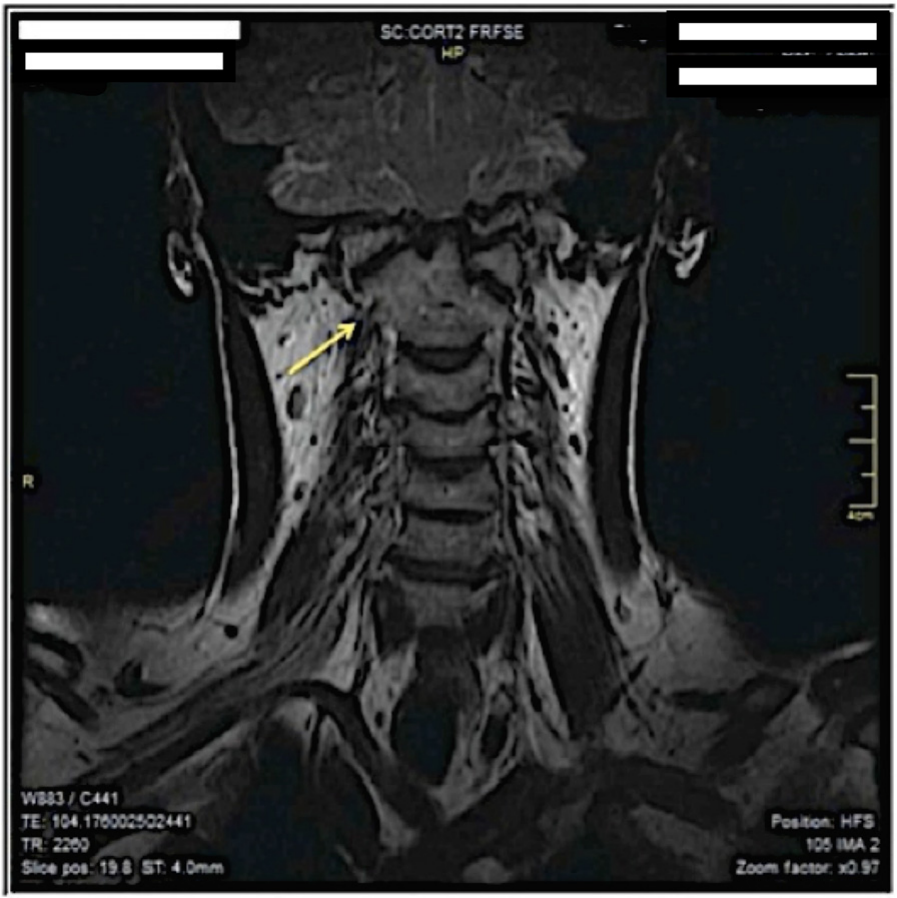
Coronal T2-weighted MRI. The MRI showing the cranial-cervical malformation with a subtotal fusion of the atlas with the occipital bone
